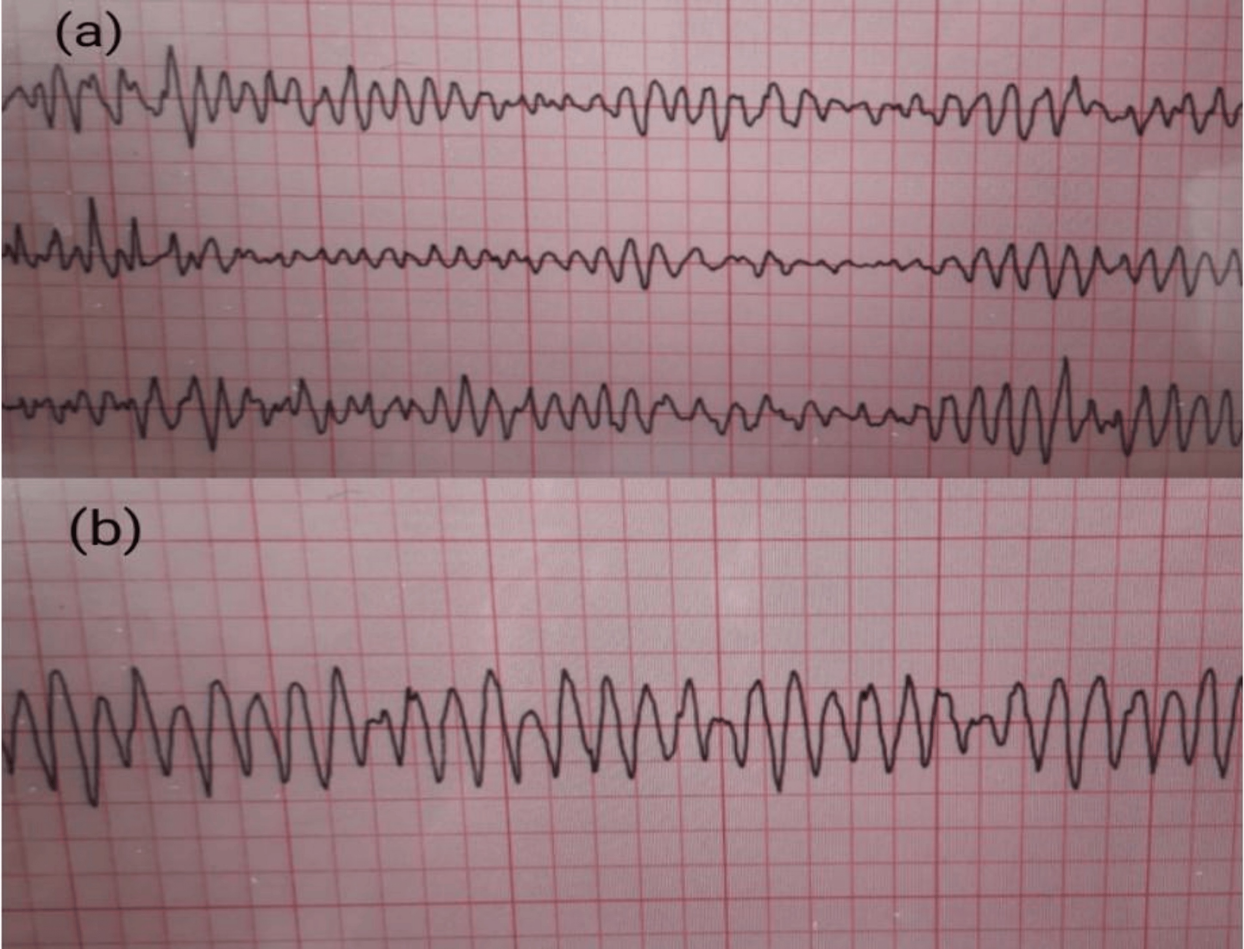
Rhythm tracing during follow-up MRI demonstrating torsades de pointes degenerating into ventricular fibrillation during the removal of the temporary pacing lead (a) Torsades de pointes. (b) Ventricular fibrillation. MRI: magnetic resonance imaging
